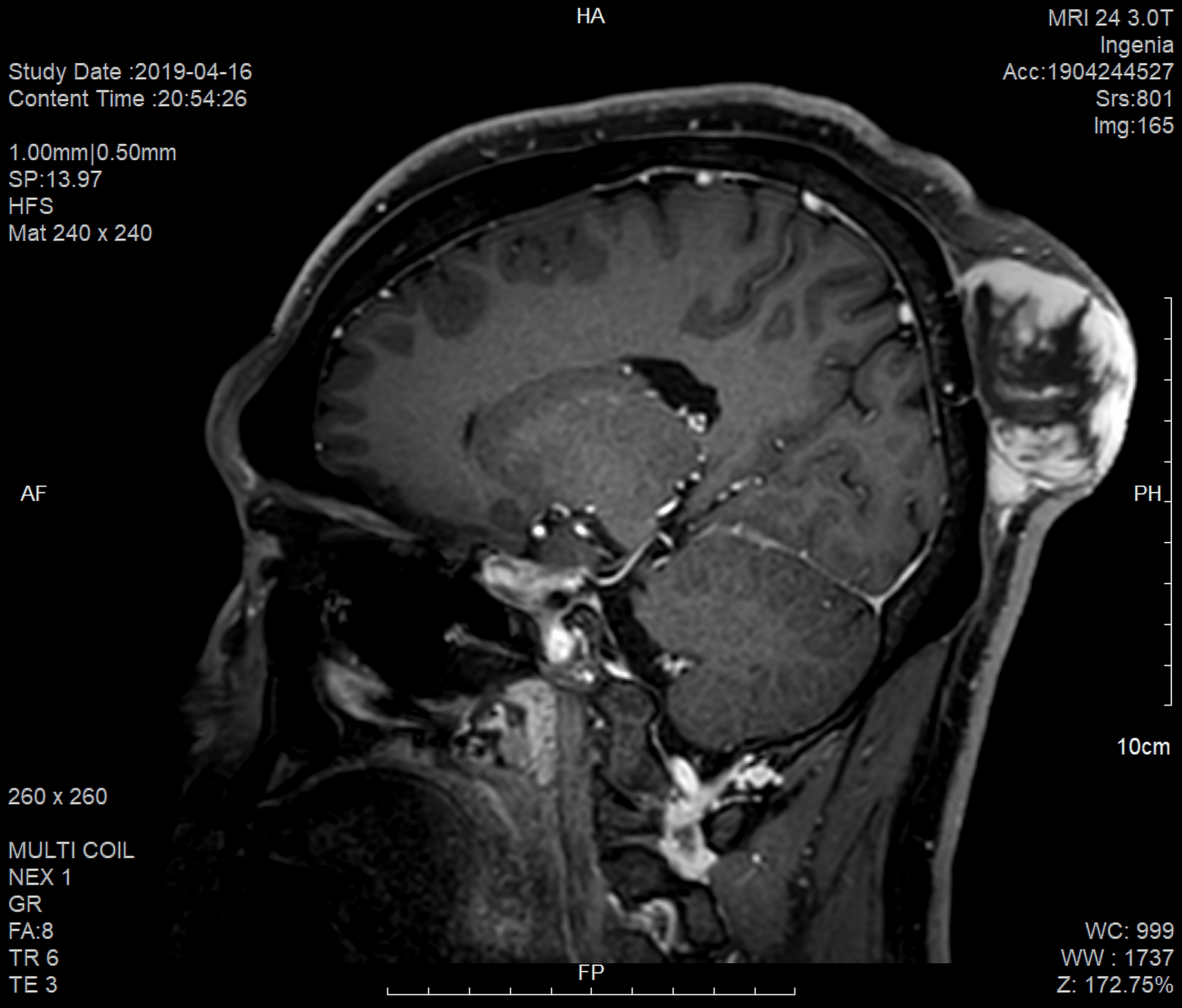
Preoperative magnetic resonance image revealing a large skin-to-periosteal tumor in the occipital area
